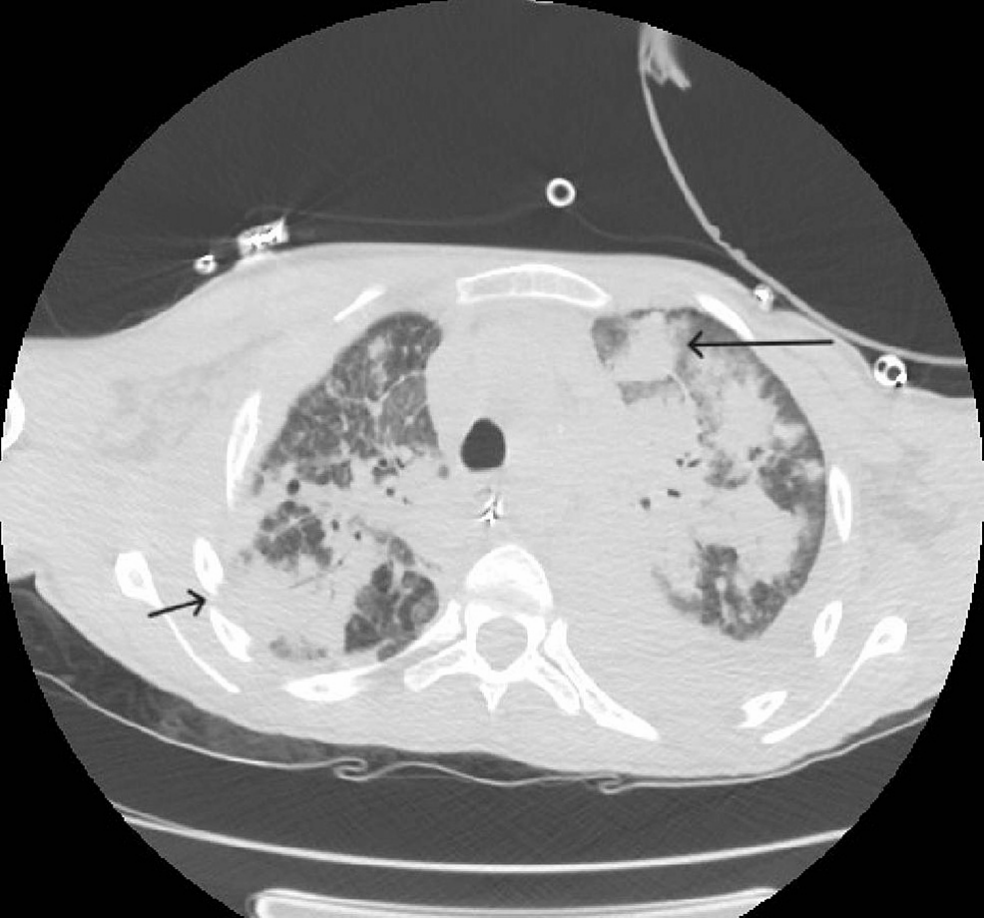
CT chest with IV contrast demonstrating pulmonary nodules surrounded by ground glass opacities (big arrow) and bony destruction of ribs (small arrow) CT: Computed Tomography, IV: Intravenous
